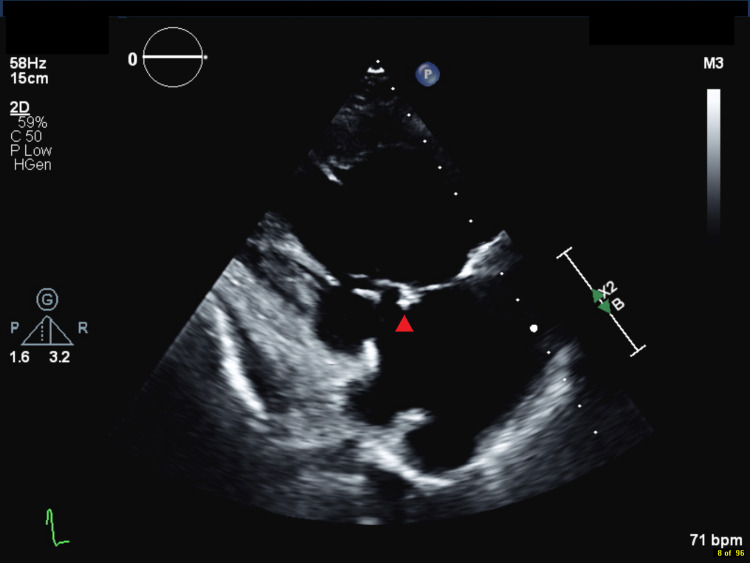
Right ventricular inflow imaging of the tricuspid valve with evidence of an independently mobile mass/vegetation (red arrowhead) on the atrial aspect of the tricuspid valve, which appears smaller in size compared to the previous echocardiogram, now measuring 7 × 6 mm.
